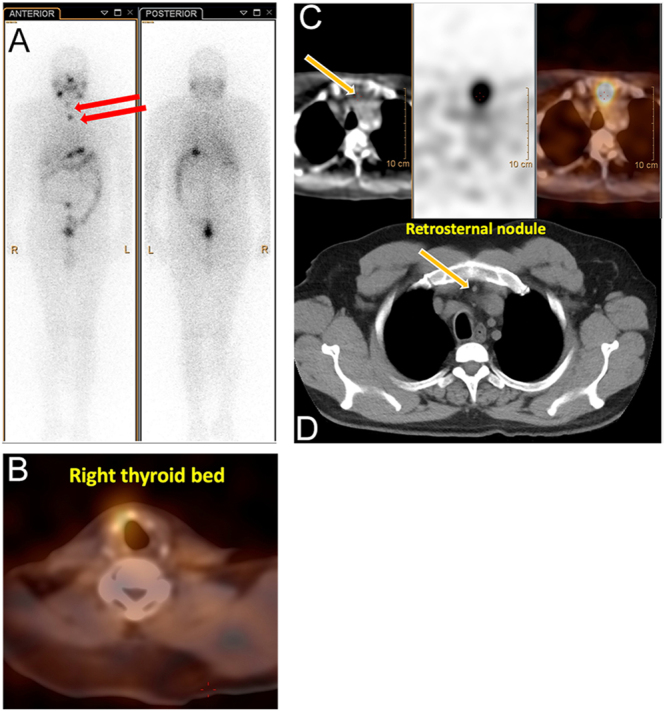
A focal area of increased radiotracer uptake is noted in the right thyroid bed and retrosternal region on whole-body images (A). These findings are more precisely localized on SPECT/CT images (B and C). Corresponding diagnostic CT performed on the same day (D) demonstrates a well-defined soft tissue nodule in the retrosternal area with superior anatomical resolution %.
